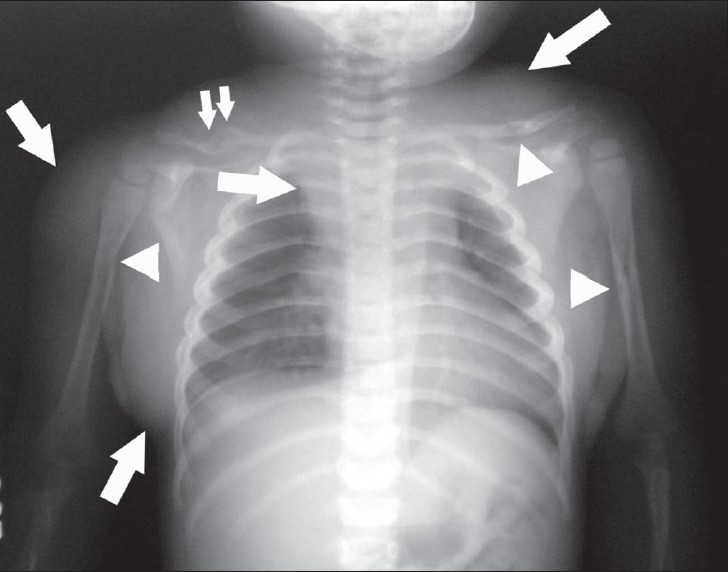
Plain radiograph of the chest and abdomen shows increased soft tissue over the shoulder and chest wall (arrows) with widening of the superior mediastinum (arrows). Cortical erosions and osteolytic areas are seen in the diaphyses of the humeri, left clavicle, and the right scapular spine (arrowheads). A healed fracture is seen in the right clavicle (double arrow)
